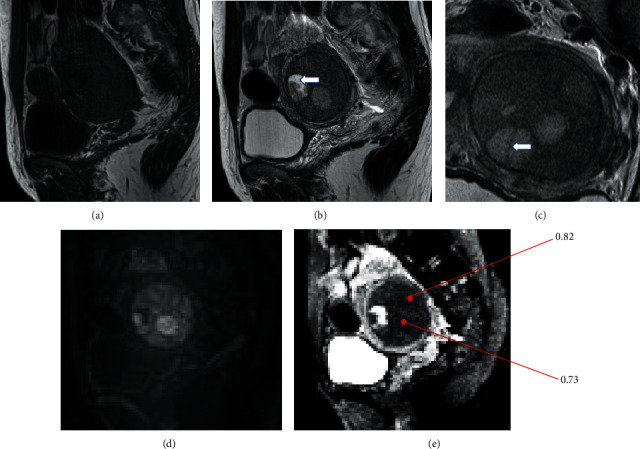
Unenhanced pelvic MRI. (a) Sagittal T1-WI shows a tumor demonstrating isointensity with the normal myometrium. (b) Sagittal T2-WI showing a tumor with a nodule-in-nodule appearance within the myometrium. The small nodules show a low signal intensity at T1-WI and a high signal intensity at T2-WI. (c) Hypointense bands within the small nodules are signalized with an arrow on coronal T2-WI. (d) At diffusion-weighted imaging (*b* = 1000 ms/mm^2^), the small nodules show a higher signal intensity than the surrounding tumors, and the surrounding tumors show a relatively higher signal intensity than the normal myometrium. (e) Both small nodules and surrounding tumors show a low signal intensity in the apparent diffusion coefficient (ADC) map. The ADC values of the small nodule and main tumor are 0.73 and 0.82 (10^−3^ mm^2^/s), respectively.
